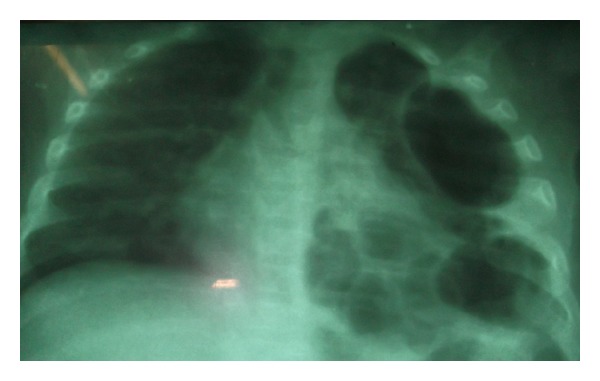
Repeat chest radiographs revealed 2 huge pneumatoceles and several smaller ones on the left lung zone.
